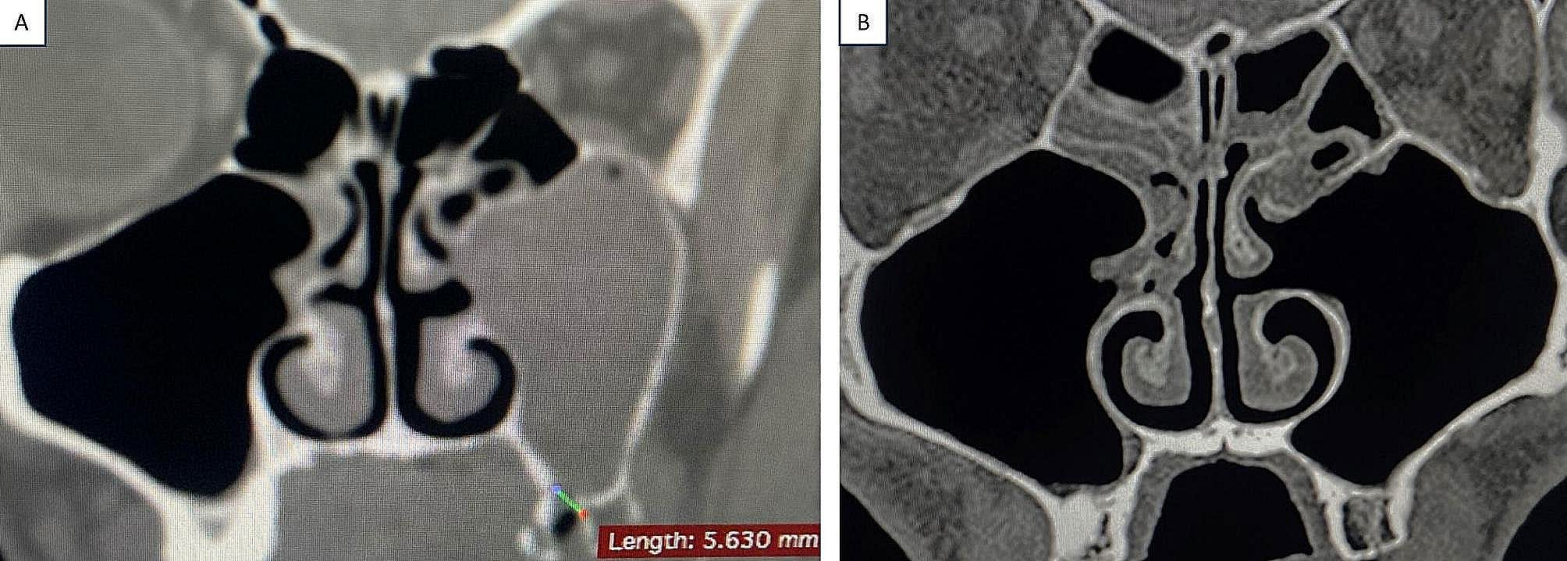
(A) Preoperative CT scan (coronal plane) showing an OAF, and discontinuity in the MS floor associated with complete opacification of the left MS. (B) Postoperative CT after 24 weeks revealed complete disappearance of the opacification at the left MS, and the fistula was obliterated
